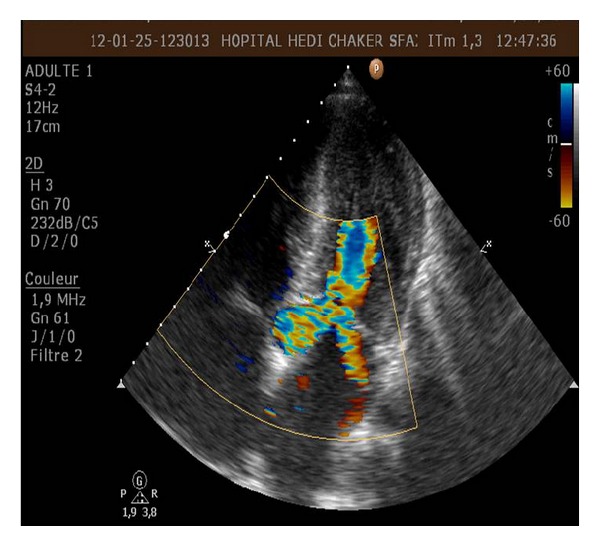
Colour flow Doppler showing evidence of high velocity turbulent flow within the midcavity of the left ventricle.
